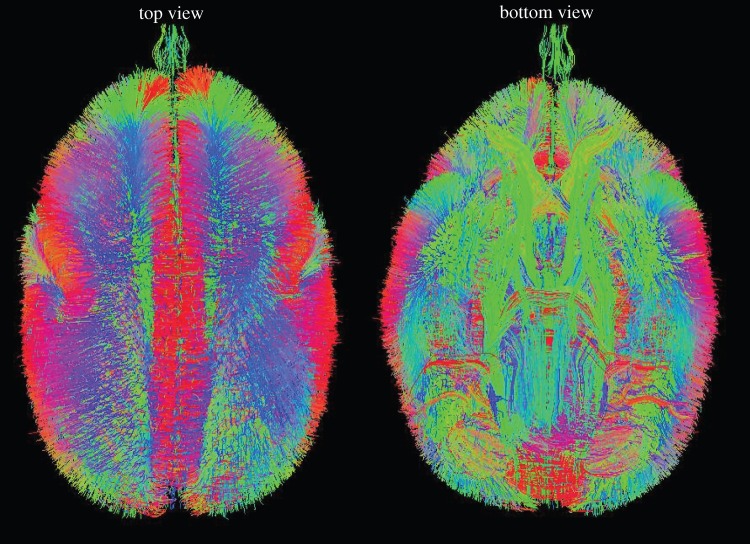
Reconstruction of whole-brain fibre structures of marmoset brain. Whole-brain tractography was reconstructed from high angular resolution diffusion MRI (HARDI) of marmoset brain and it enables analysis of the structural connectivity between remote anatomical regions in macro scale.
